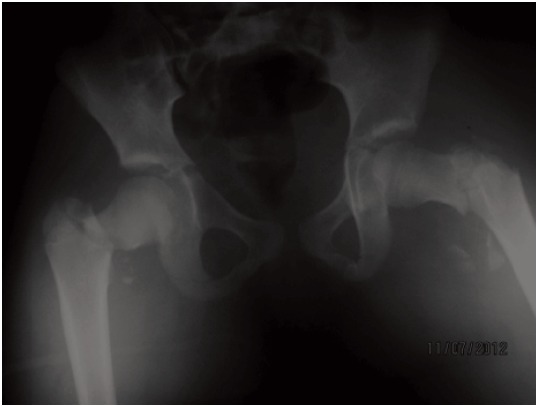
Anterioposterior radiograph of pelvis showing bilateral
